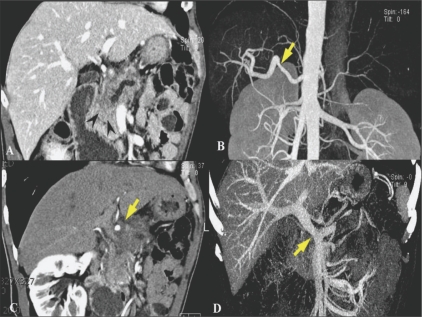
A 68-year-old man with pancreatic cancer. Oblique coronal MPR CT scan image (A) shows an ill-defined infiltrating mass (arrowheads) in the pancreatic head causing upstream pancreatic duct dilatation. Oblique coronal MPR (B) and MIP (C) arterial phase CT scan images demonstrate circumferential soft tissue infiltration around the common hepatic artery with deformity (arrows) suggesting tumor invasion. Coronal portal venous phase MIP image (D) reveals marked narrowing of the main portal vein near the portomesenteric confluence (arrow), indicating tumor invasion into the main portal vein in one plane. MPR : multiplanar reconstruction, MIP : maximum intensity projection
